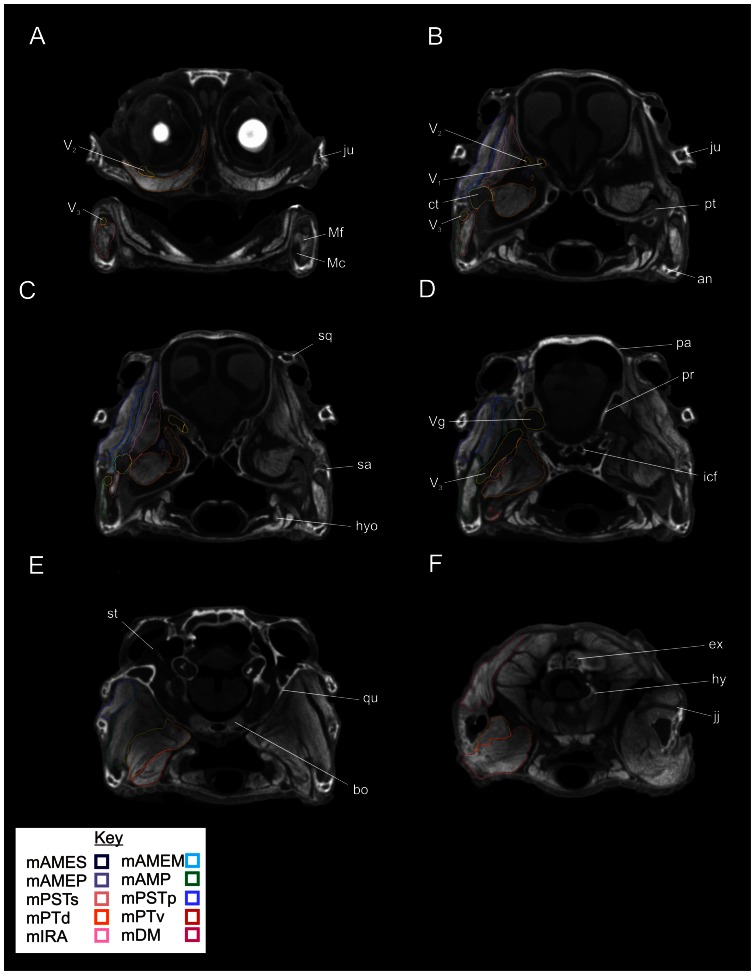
Rostral views of axial sections through the segmented CT data showing labeled individual right jaw muscles on the left, and unlabeled left structures on the right. A, Slice 391/911; B, Slice 316/911; C, Slice 305/911; D, Slice 283/911; E, Slice 240/911; F, Slice 154/911. Abbreviations: an, angular; bo, basioccipital; ct, cartilage transiliens; ex, exoccipital; hyo, hyoid; icf, internal carotid foramen; jj, jaw joint; ju, jugal; Mc, Meckel's cartilage; Mf, Meckelian fossa; naa, neural arch of atlas; pa, parietal; pr, prootic; pt, pterygoid; qu, quadrate; sa, surangular; sq, squamosal; st, stapes; V_1_, ophthalmic nerve; V_2_, maxillary nerve; V_3_, mandibular nerve; Vg, trigeminal ganglion.
